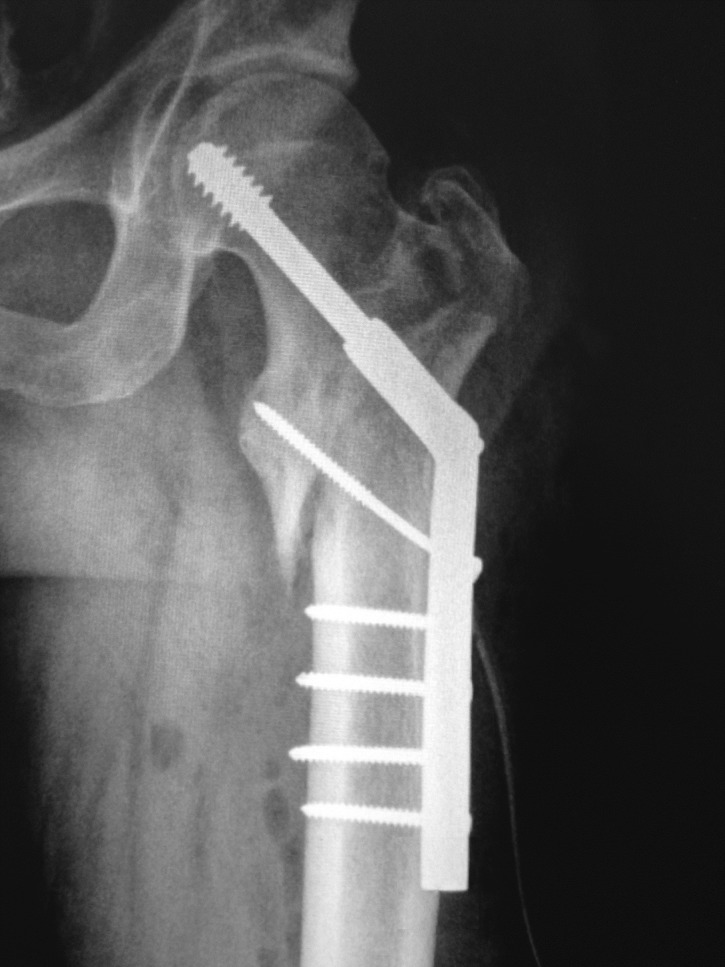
Postoperative antero-posterior X-ray view revealing fracture and dislocation reduction and fixation with dynamic hip screw
